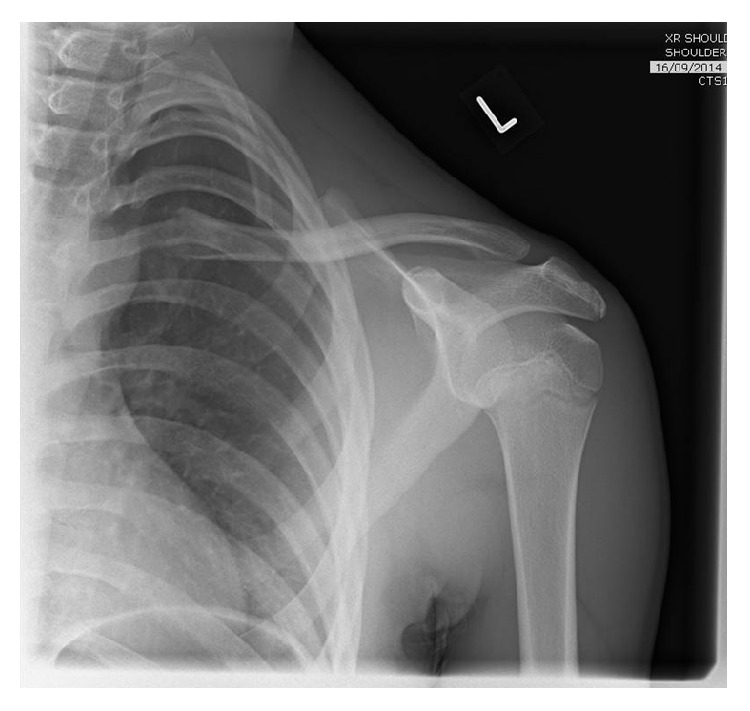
AP X-ray indicating minimally displaced mid-clavicle fracture.
